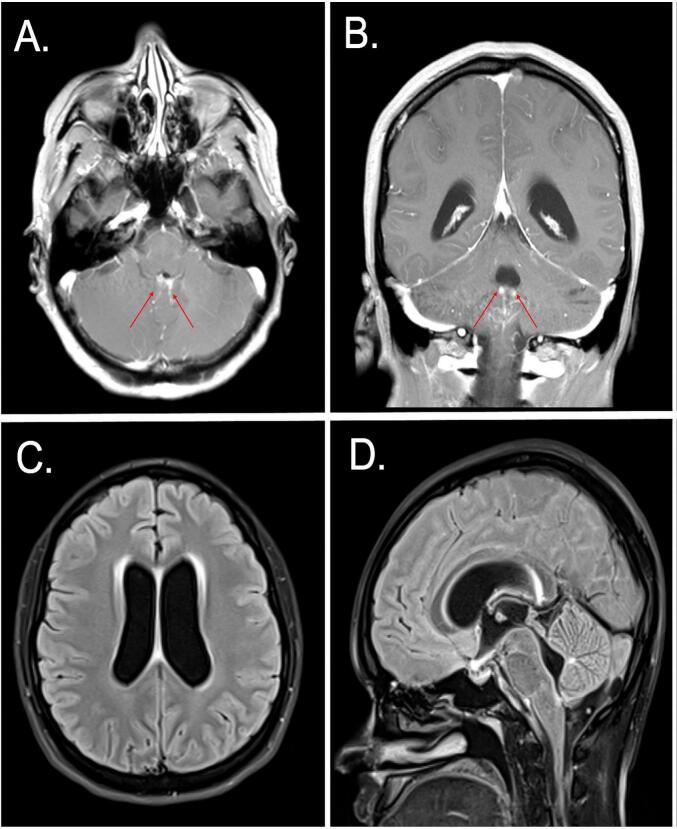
MRI of the brain revealing nodular enhancement in the brainstem (red arrows: A, B) and communicating hydrocephalus (C, D). *MRI*, magnetic resonance imaging. (For interpretation of the references to colour in this figure legend, the reader is referred to the web version of this article.)
